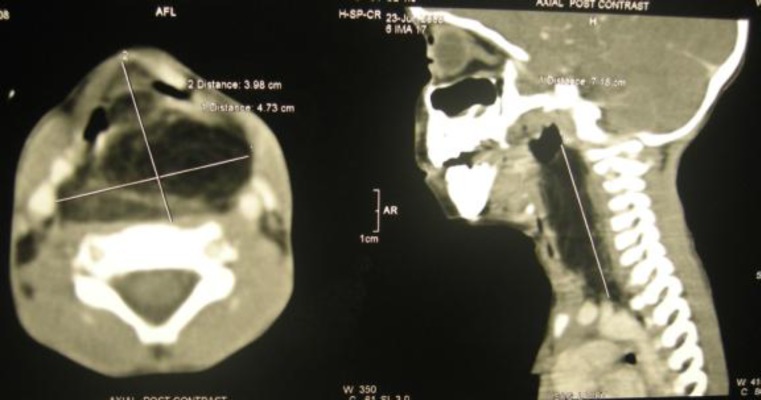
Reconstructed Contrast enhanced Computed tomography (Axial and Sagittal section) showing a fat attenuating mass of 3.98X4.7X7.0 cm in the retropharynx from C1 to D2, displacing the larynx anteriorly and bilateral and displacing the carotid laterally
